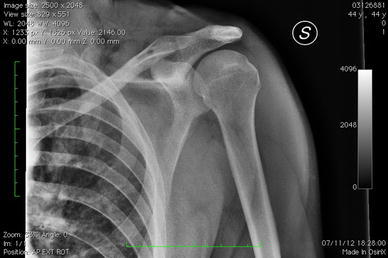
Postoperative X-rays: left AC joint stabilized with the biological graft
